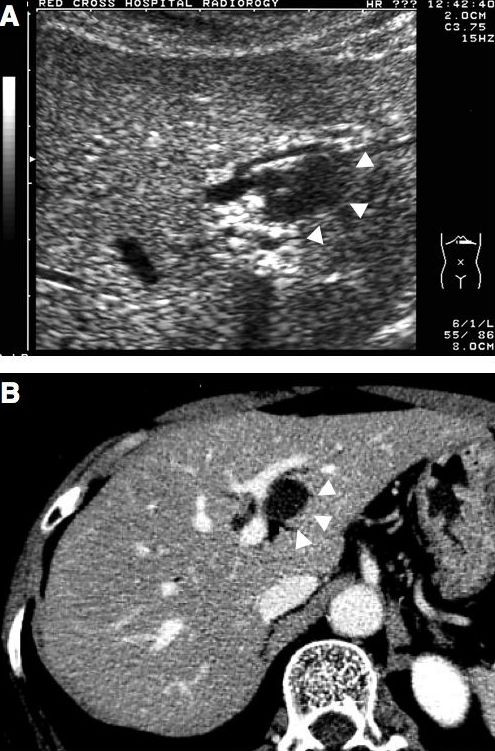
Abdominal ultrasonography (A) and computed tomography (B) show a cystic lesion measuring 2.0 cm in maximal diameter at the left lateral segment of the liver with peripheral left lateral anterior subsegmental bile duct (B3) dilatation. Arrows head indicate the cystic lesion.
